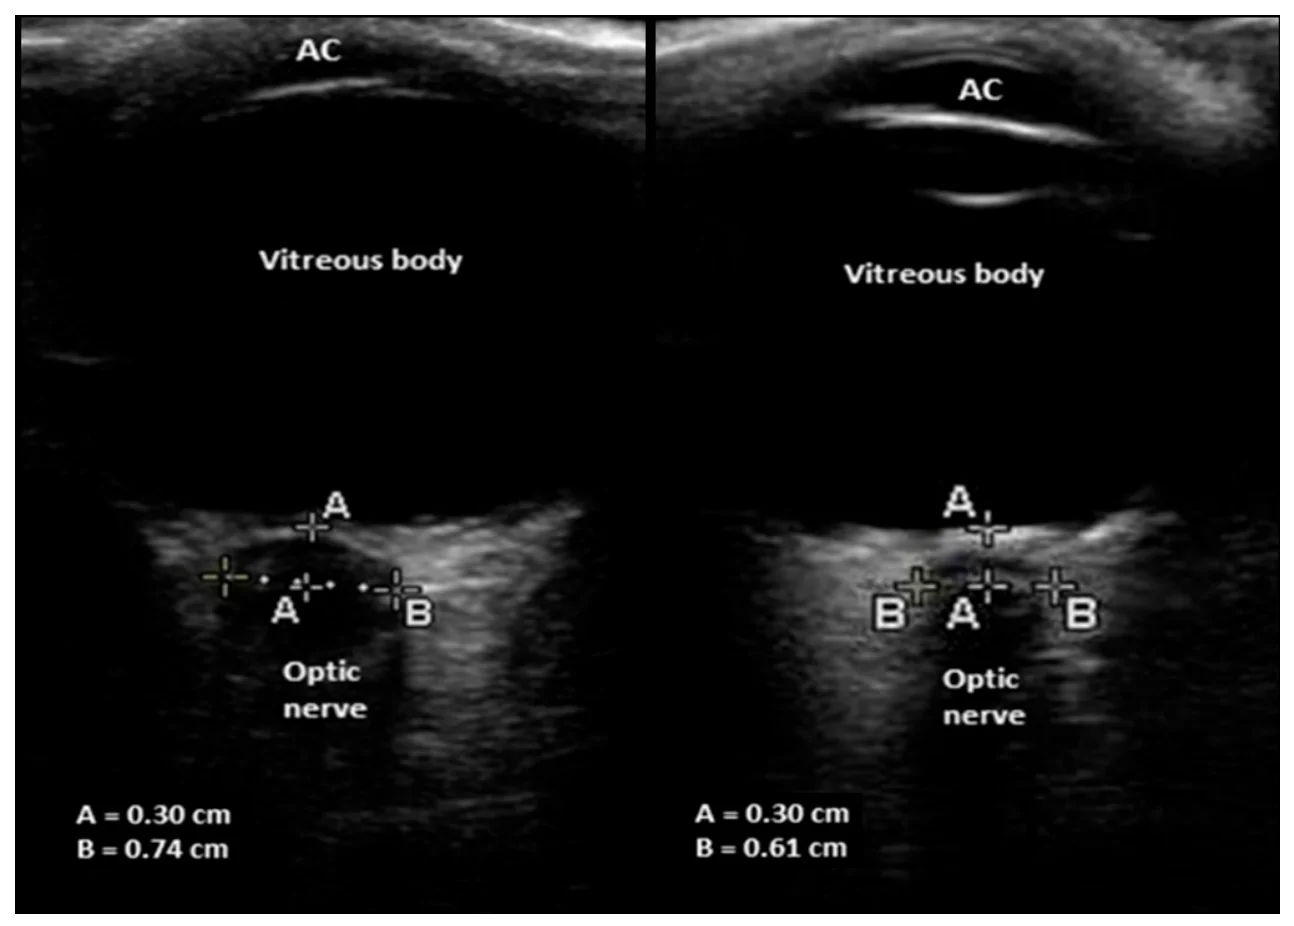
Optic nerve ultrasound revealing a dilated optic nerve sheath of 0.74 cm in a spontaneous CSF leak patient the day after closure of the defect (left). Six hours after administration of 500 mg of acetazolamide, the patient has an optic nerve sheath diameter of 0.61 cm and a drop in CSF pressure of 14 cm H20 according to lumbar drain measurements (right).
